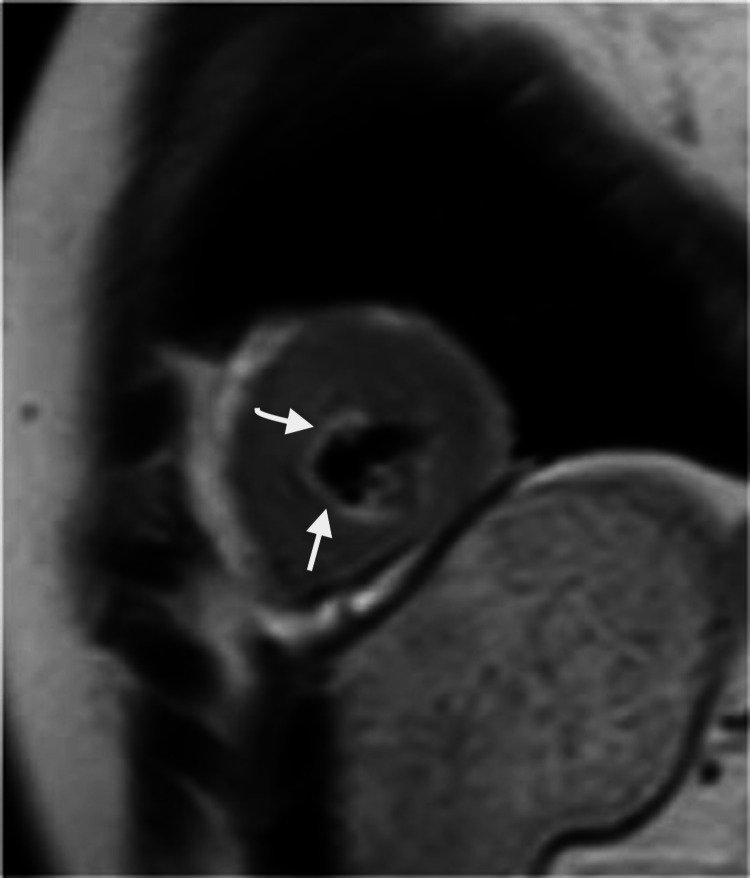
Gadolinium contrast-enhanced cardiac MRI showing subtle mid-septal to apical septal and inferior wall linear endocardial enhancement (white arrow). MRI, magnetic resonance imaging
